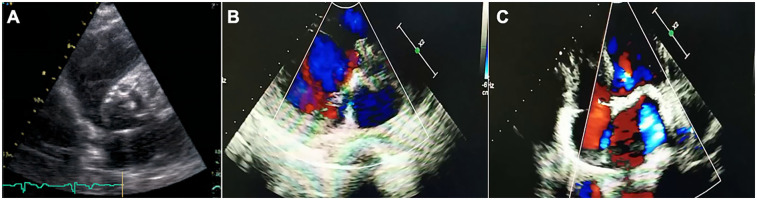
Transthoracic echocardiographic assessment of aortic stenosis in the proband. (A) Parasternal short-axis view showing tricuspid aortic valve with markedly thickened, calcified leaflets (arrows) and restricted systolic opening. (B,C) Apical five-chamber views with color Doppler demonstrating high-velocity, turbulent systolic flow (mosaic pattern) across the stenotic valve, consistent with severe obstruction.
